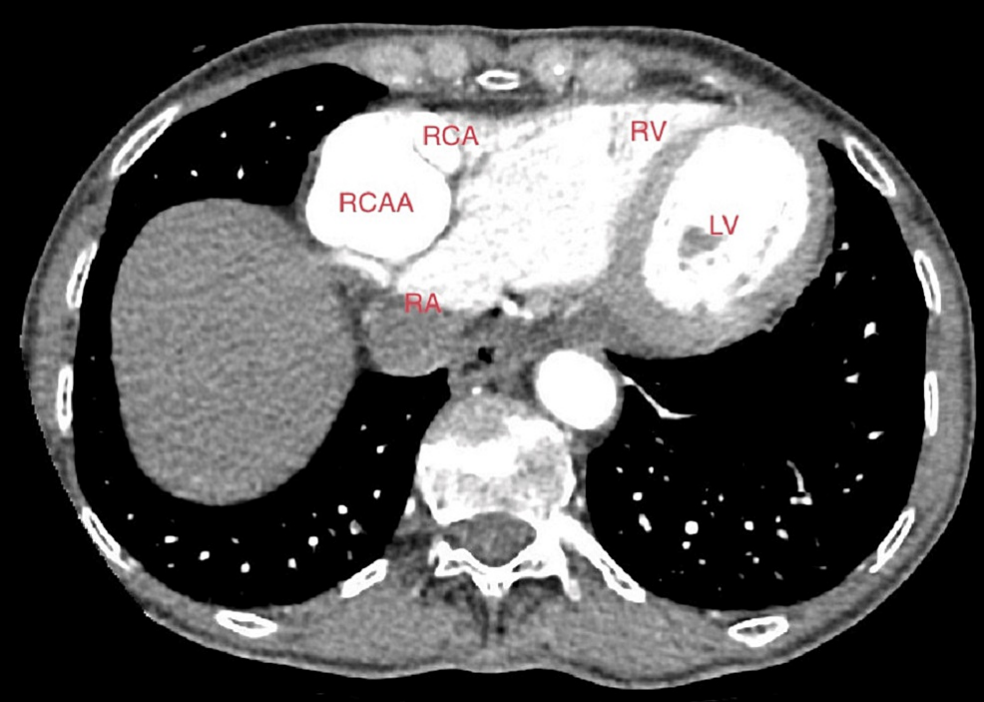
Coronary CTA demonstrating distal RCAA. Image obtained from the patient’s chart. CTA: computed tomographic angiography; RCAA: right coronary artery aneurysm; RCA: right coronary artery; RV: right ventricle; LV: left ventricle; RA: right atrium
